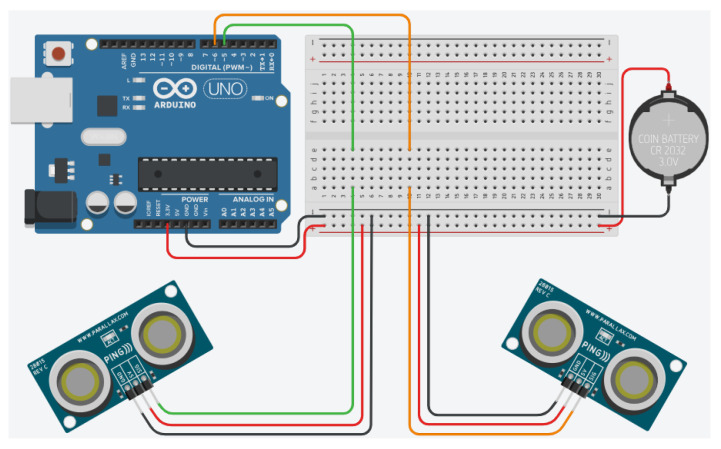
Setup of the two ultrasonic sensors used to triangulate the position. Wires indicated in red are connected to Vcc (+5V) and black are connected to ground, while other colors are signals (0–5 V).
